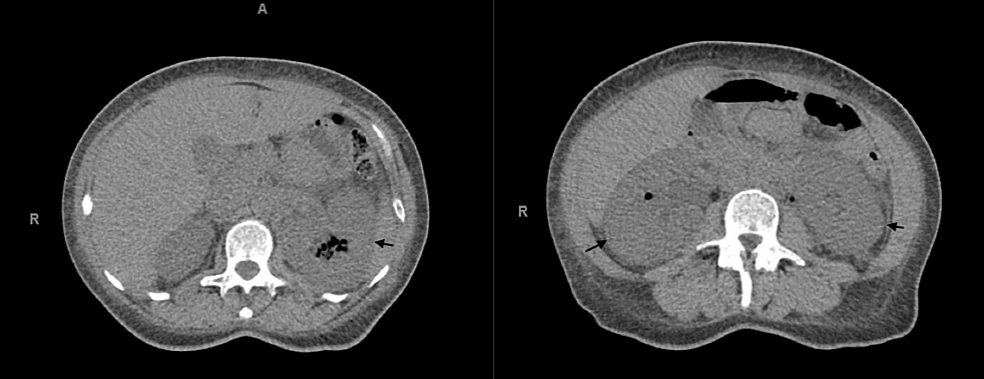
Non-contrast axial CT of abdomen and pelvis showing bilateral emphysematous pyelonephritis. Bilateral kidneys are enlarged with multiple foci of air in the bilateral kidneys, left greater than right.
